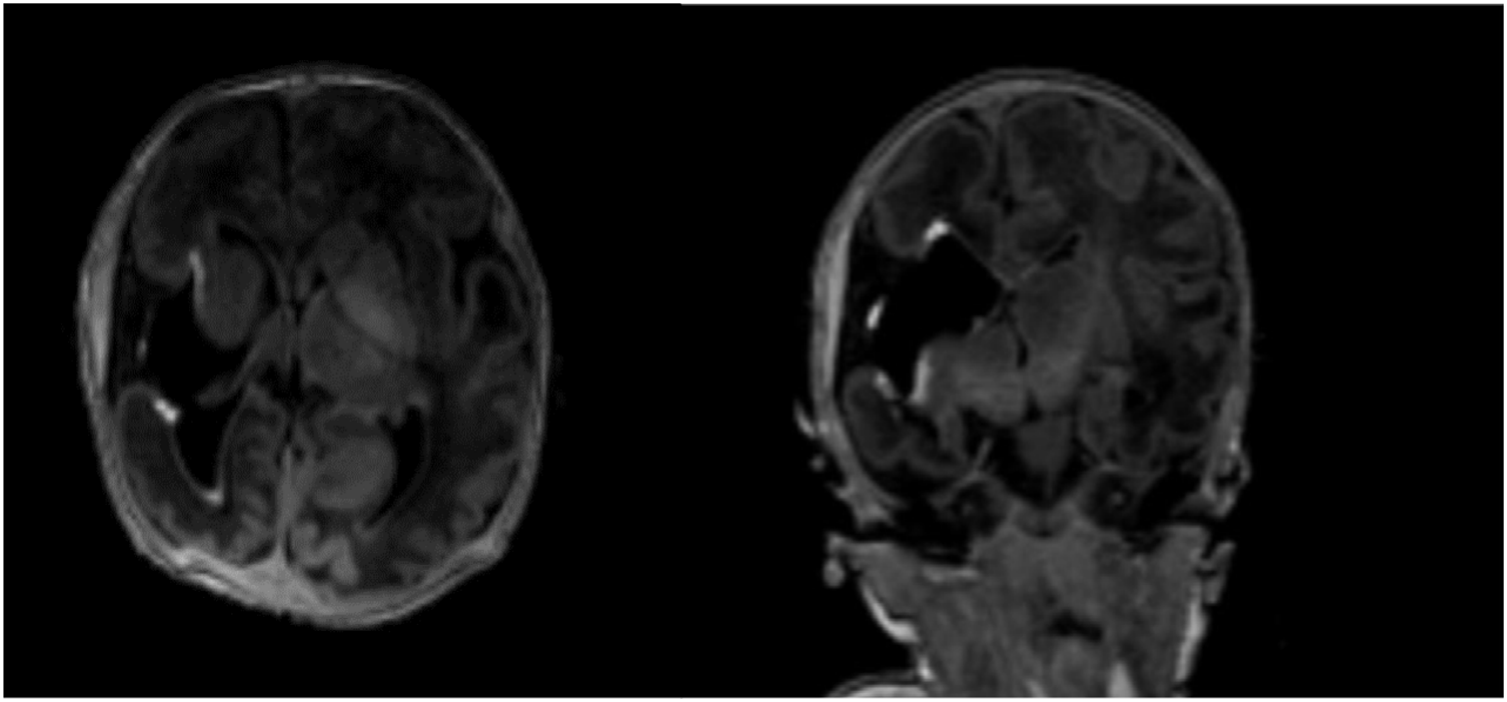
Cerebral MRI performed after birth. Axial and coronal MRI T1 sequences showing extensive poroencephalic area in the right front-temporal and insular zone with “ex vacuo” enlargement of the right lateral ventricle, reduced volume of the right cerebral hemisphere. Abnormal development of the middle-lower right frontal cortex with a polylobate appearance due to reduced furrows width and increased number of turns confirming the micropolygyria extended up to the right parietal lobe.
